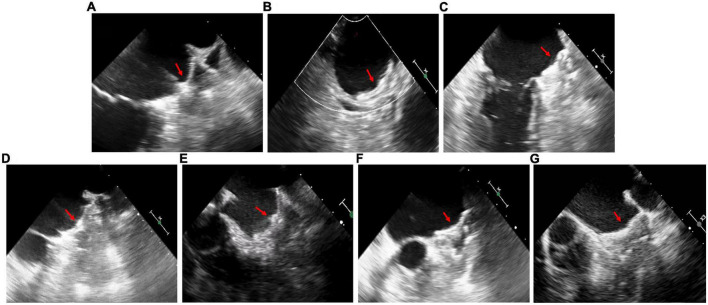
Transesophageal echocardiography images in patient (A–G) after left atrial appendage closure. The red arrows point to devices.
